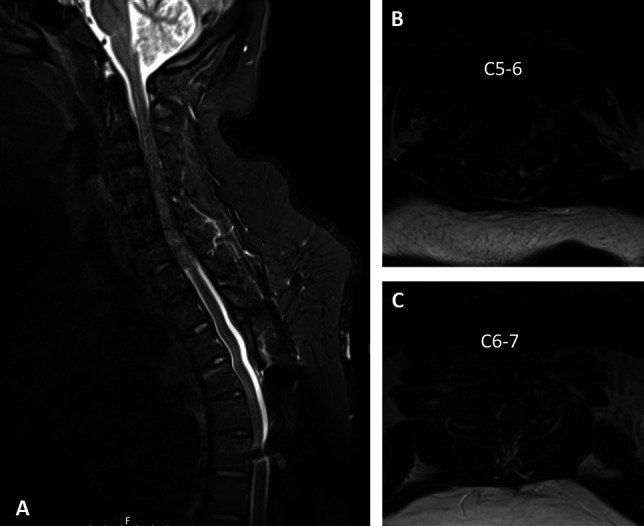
Radiographs showing sagittal short tau inversion recovery magnetic resonance imaging of the cervical and thoracic spine (A) and T2 axial cross sections at C5-6 (B) and C6-7 (C) demonstrating recurrent epidural mass from C4-T1.
